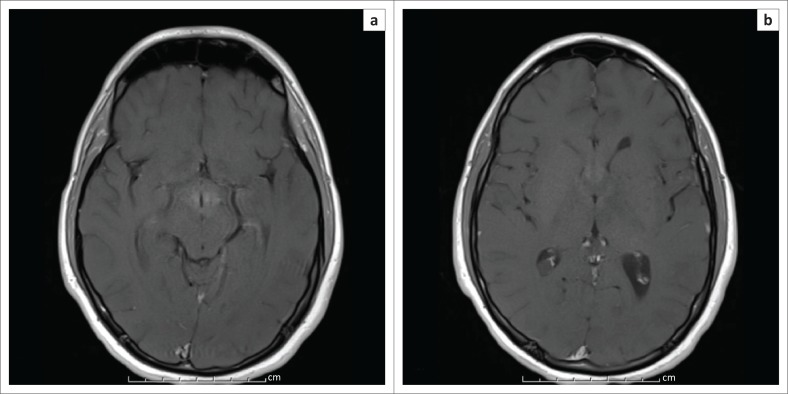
T1-weighted post-contrast imaging (a and b) following surgery demonstrates near-complete resolution of the previously noted enhancement.
